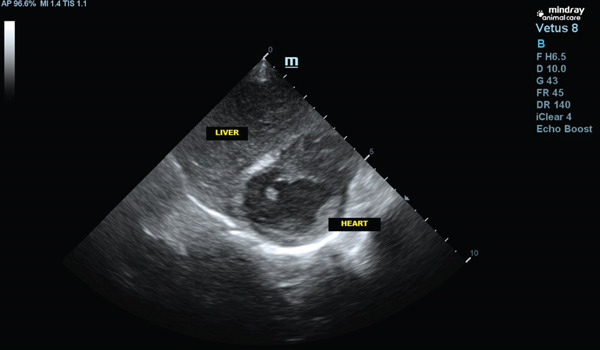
Thoracic ultrasound showing anomalous relationship between the left medial and lateral liver lobes and the pericardium.
